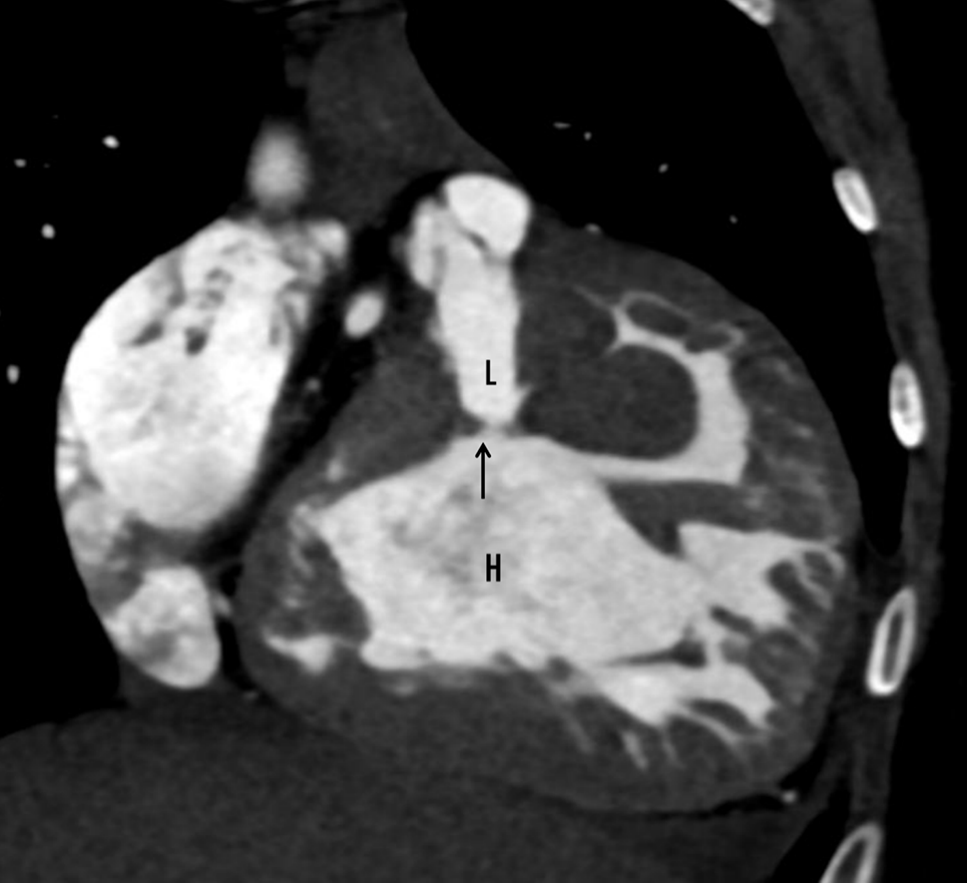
Cardiac CT coronal section showing thickened right ventricular wall with an anomalous band (thin arrow) running beneath the infundibulum of the outflow tract, dividing the ventricle into high (H) and low (L) pressure chambers
